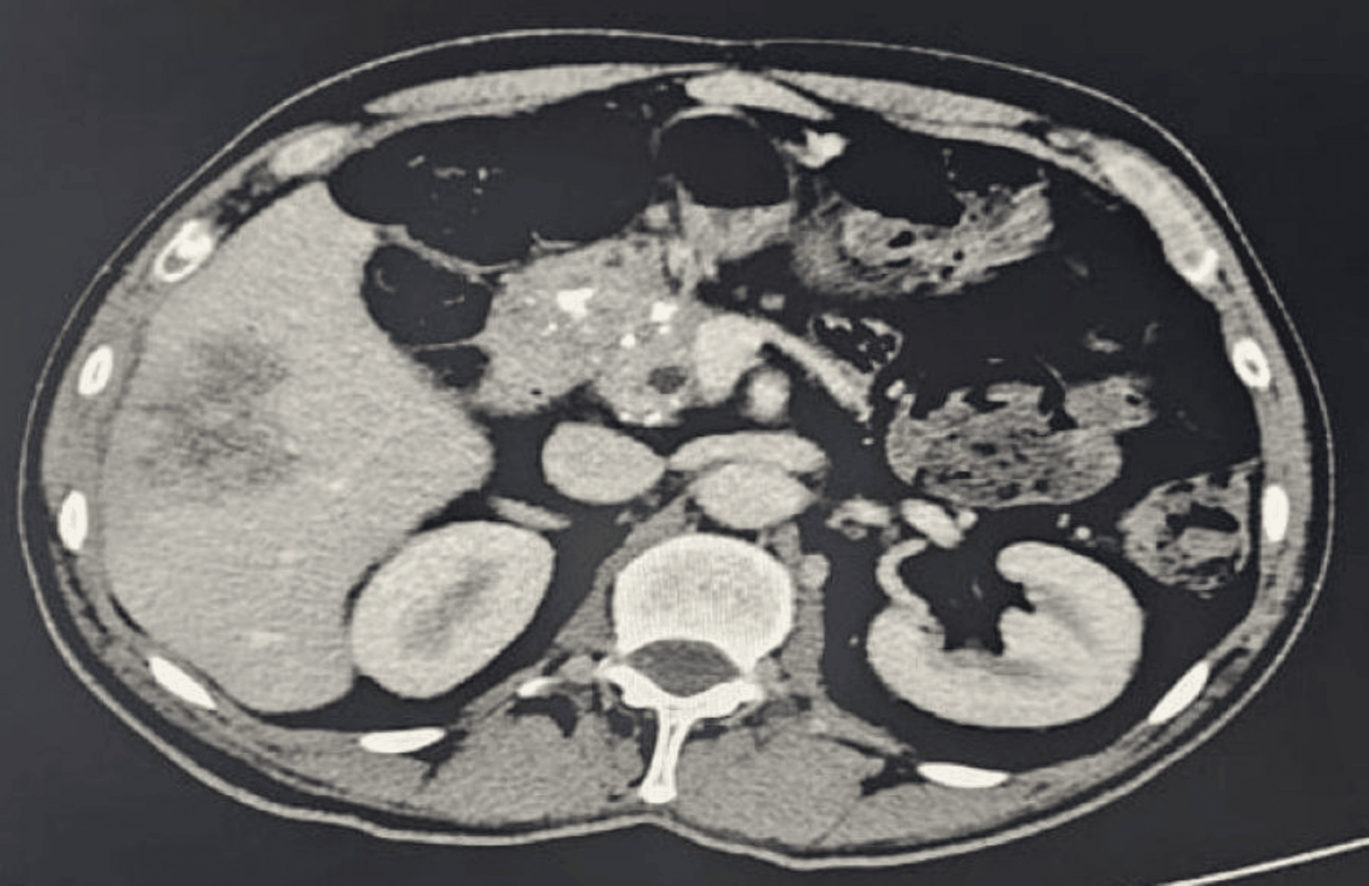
Contrast-enhanced CT after four months of treatment showed a reduction of the hepatic lesion measuring about 28 x 20 x 15 mm
